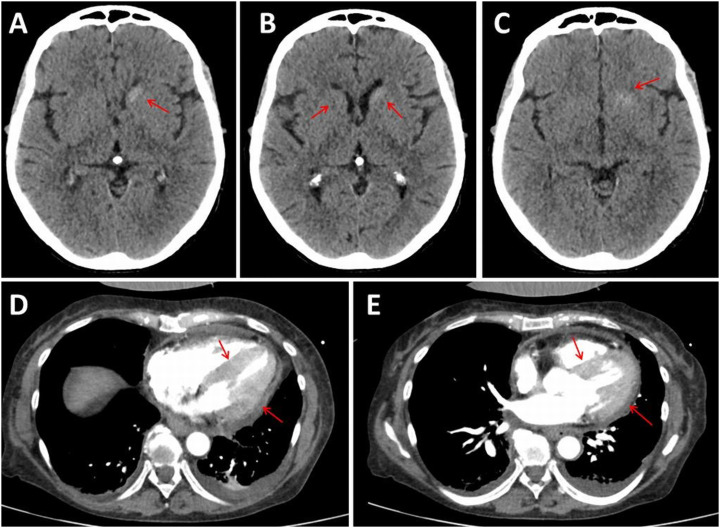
Cerebral CT (axial view) showing mild hyperdensities lateral and caudal to the left lateral ventricle/caput nuclei caudati ‒ possibly representing calcifications, hemorrhage or CNS involvement in WM (BNS), without significant change compared to a previous finding (panels A, B, C). Thoracic CT showed marked thickening of the left ventricular myocardium and pericardial effusion (panels D, E).
